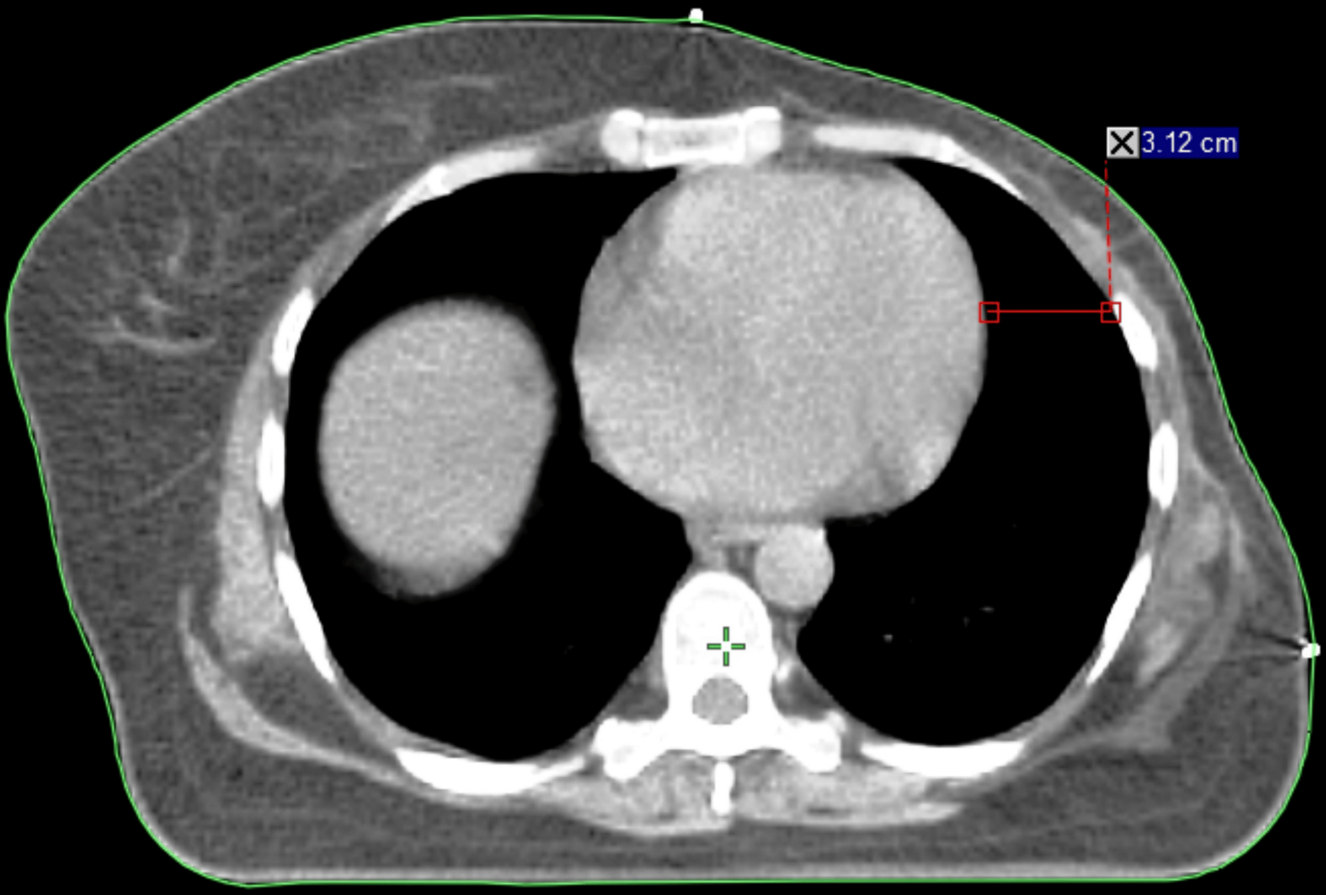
CT image acquired during FB. This axial CT shows that the heart-chest wall distance is reduced to 3.12 cm. The heart lies closer to the left chest wall and within the high-dose region, underscoring the need for heart-sparing techniques in left-sided breast radiotherapy. FB: free breathing
